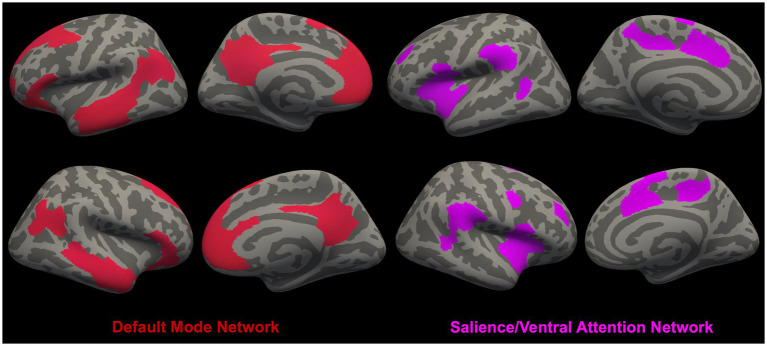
Overview of brain networks [i.e., Default Mode Network (DMN) (79) and Salience/Ventral Attention Network (SN/VAN) (80)] (81) that are most commonly found to be involved in functional MRI and brain morphometry research of suicidal thoughts and behavior.
